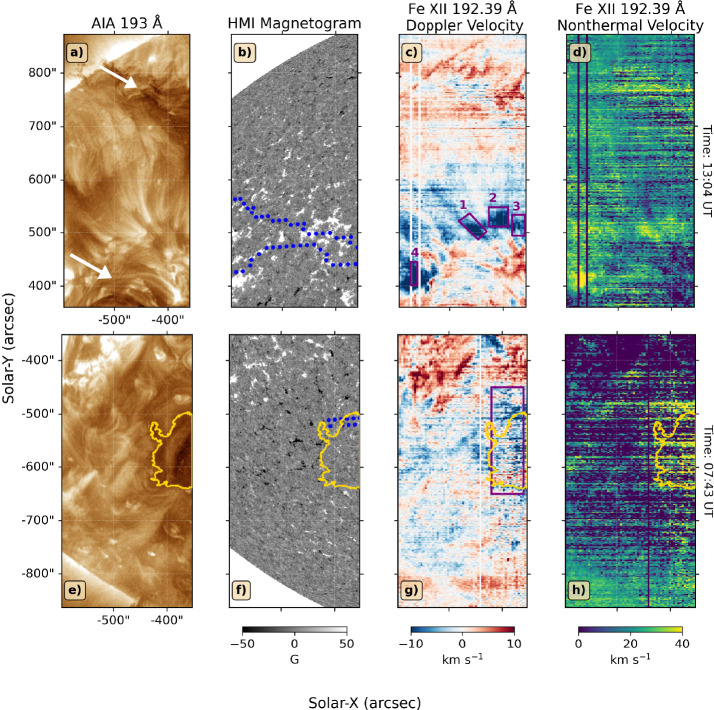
Plasma diagnostics of the low solar corona at the open-field corridor (EIS North FOV, top row) and CH (EIS South FOV, bottom row). From left to right, the columns show AIA 193-Å observations, HMI LOS photospheric magnetograms, EIS Fe xii 192.39 Å Doppler velocity maps and EIS Fe xii 192.39 Å nonthermal velocity maps. The yellow contours indicate the CH boundaries, and the dotted blue contours outline the open-field locations. The purple boxes indicate the regions where we average the spectra to obtain plasma properties shown in Table 1. The colour scale of the Doppler velocity maps was set to [-10,10] km s^−1^. The nonthermal velocity maps are saturated at [0,40] km s^−1^. HMI magnetograms are saturated at $\pm 50$ G.
